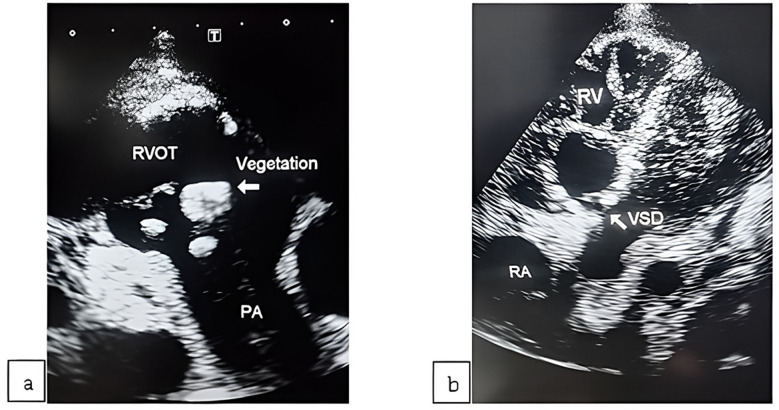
(a) Initial transthoracic echocardiogram. (b) TTE; after five days. RVOT= Right ventricular outflow tract. PA= Pulmonary artery, RA= Right atrium, RV=Right ventricle, VSD= Ventricular septal defect.
